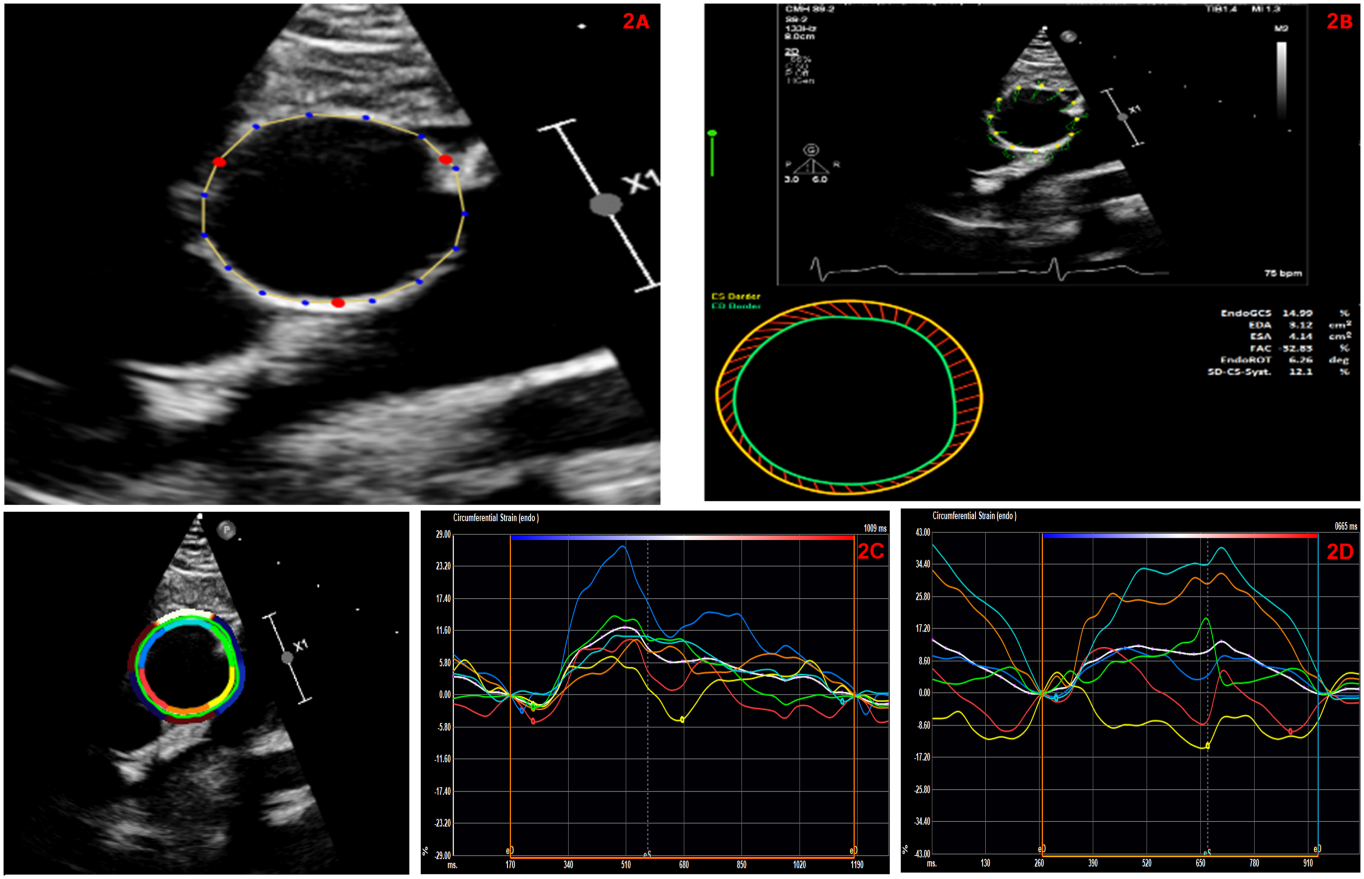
(A) Automated contour tracing to track the inner border of the ascending aortic wall using parasternal short axis view. (B) Automated global circumferential strain calculated by averaging the peak circumferential strains of the 6-segmented aortic model and expressed as an absolute value. (C) Representative AAo-GCS curves in a patient with repaired conotruncal abnormality. This patient had a AAo-GCS of 7.6%. (D) Representative AAo-GCS curves in an age and gender matched healthy control. This patient had a Aao-GCS of 12.7%.
